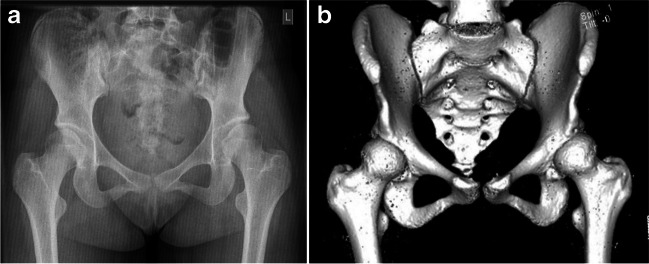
Pre-operative imaging: a plain AP view radiograph of a patient with acetabular dysplasia and b CT scan with 3D reconstruction demonstrating the extent of femoral head undercoverage
